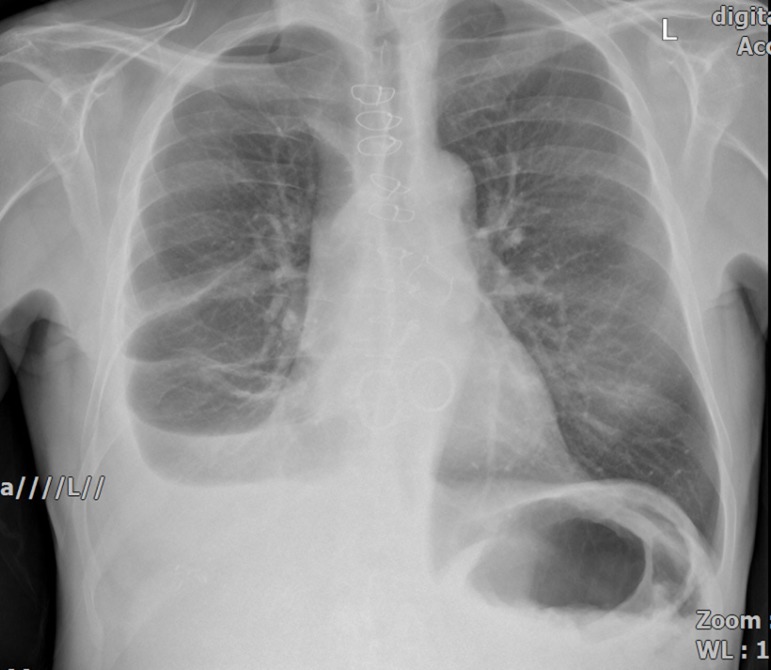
Chest X-ray showing all four replaced valves.
